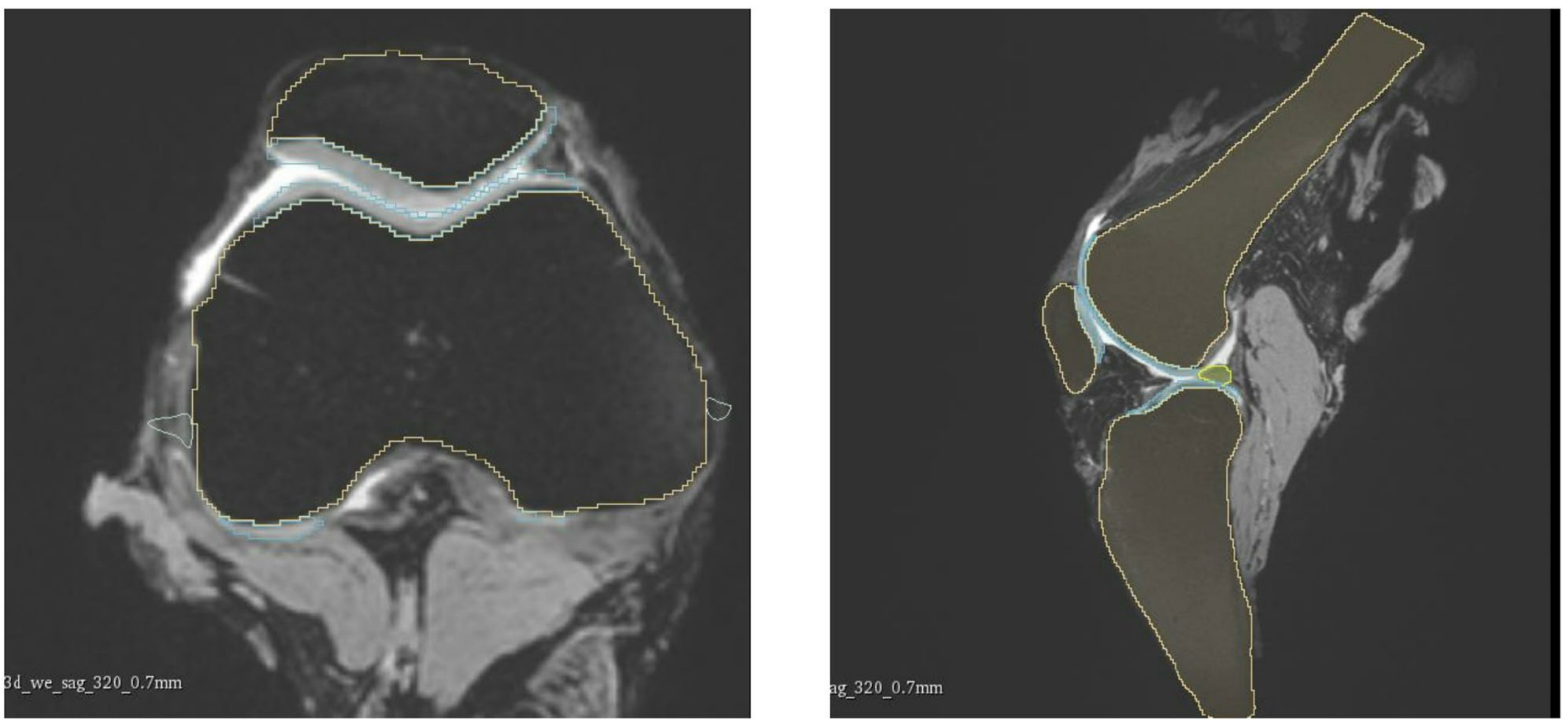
Segmentation of the MRI scans using 3D Slicer, obtaining relevant osseous and cartilaginous structures
